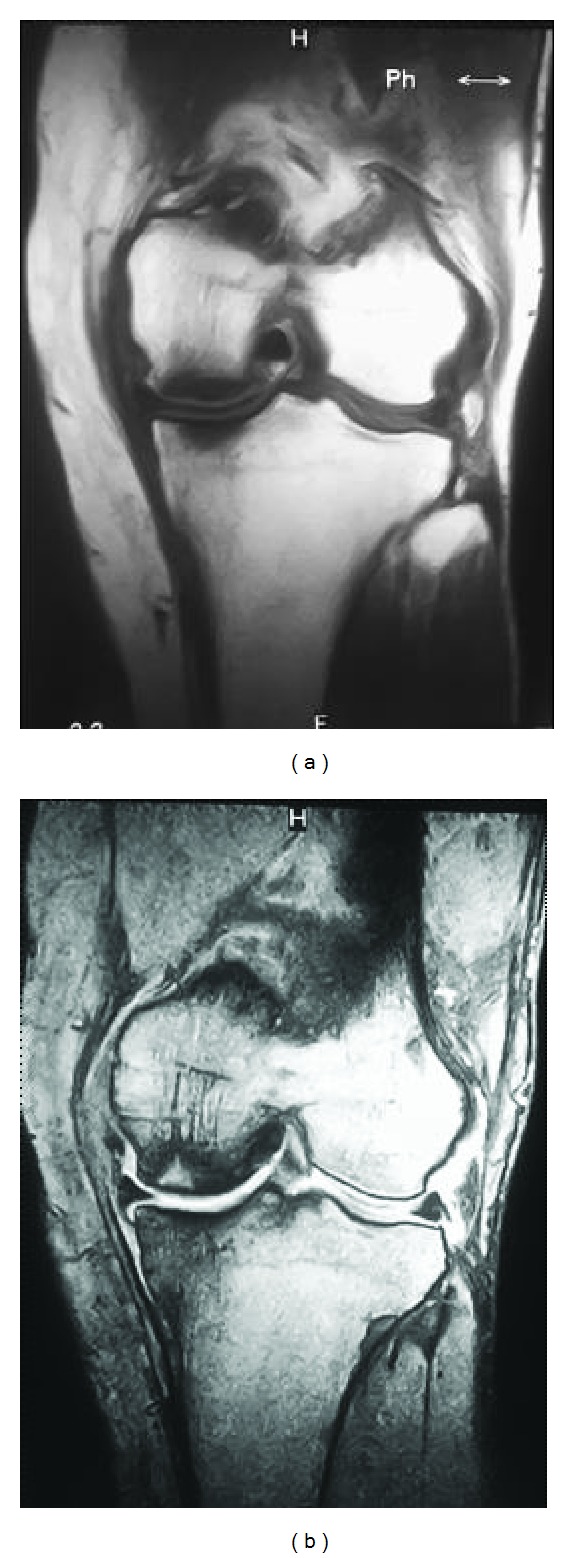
Case 1. Preoperative magnetic resonance images of the affected knee. T1-weighted image shows low signal intensity of the medial femoral condyle and tibial plateau (a). T2-weighted image shows high intensity in the central area of the femoral lesion and the surrounding low-intensity area (b).
